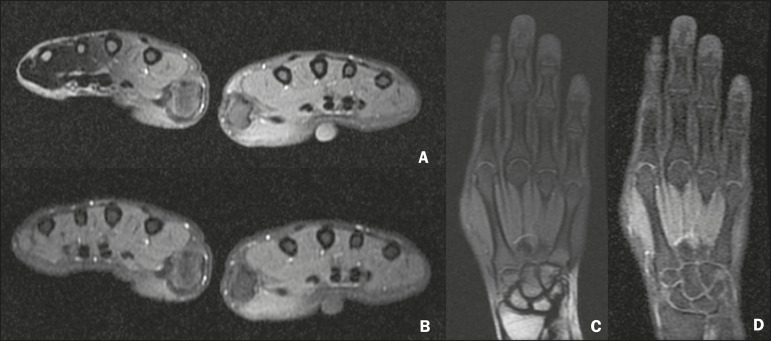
Short- and long-axis images of the hands and wrists of a 30-year-old female patient with psoriatic arthritis followed by a protocol for rheumatologic disease with simultaneous contrast-enhanced imaging of both hands. Contrast-enhanced T1-weighted FSE sequence with fat suppression (A,C) and contrastenhanced T1-weighted IDEAL sequence (B,D), demonstrating that the latter (the Dixon technique) provided more effective fat suppression in the hand and wrist.
